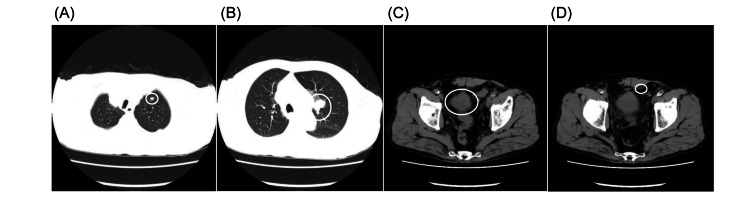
CT scan of the chest and abdomen. Circles indicate (A) lung metastasis, (B) mediastinal lymph node metastasis, (C) primary prostate cancer, and (D) peritoneal dissemination.
